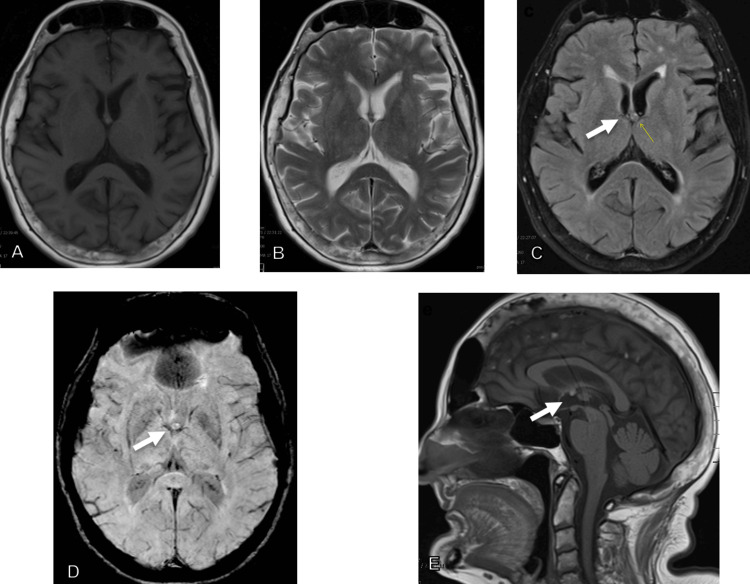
MRI brain study that depicted a colloid cyst (white arrows) that was not reported (A) T1 axial. (B) T2 axial. (C) FLAIR axial. (D) SWI axial demonstrating the black rim susceptibility sign. (E) T1 sagittal.
